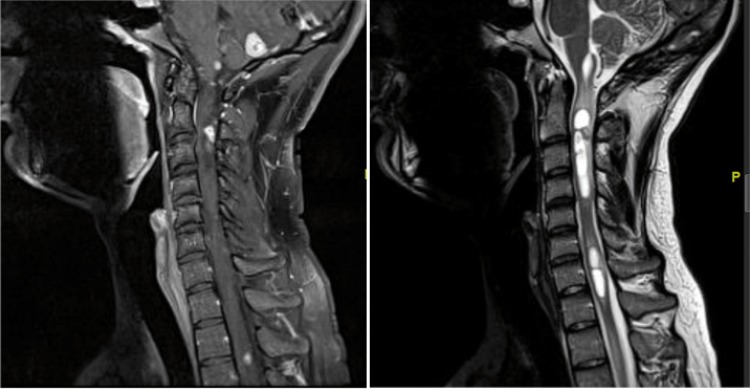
Sagittal MRI of posterior fossa and cervical spine. *Left*: T1 with gadolinium shows multiple enhancing HB tumors involving cerebellum, brainstem, and spinal cord. *Right*: T2 MRI shows multiple intramedullary cystic lesions consistent with VHL hemangioblastoma.
